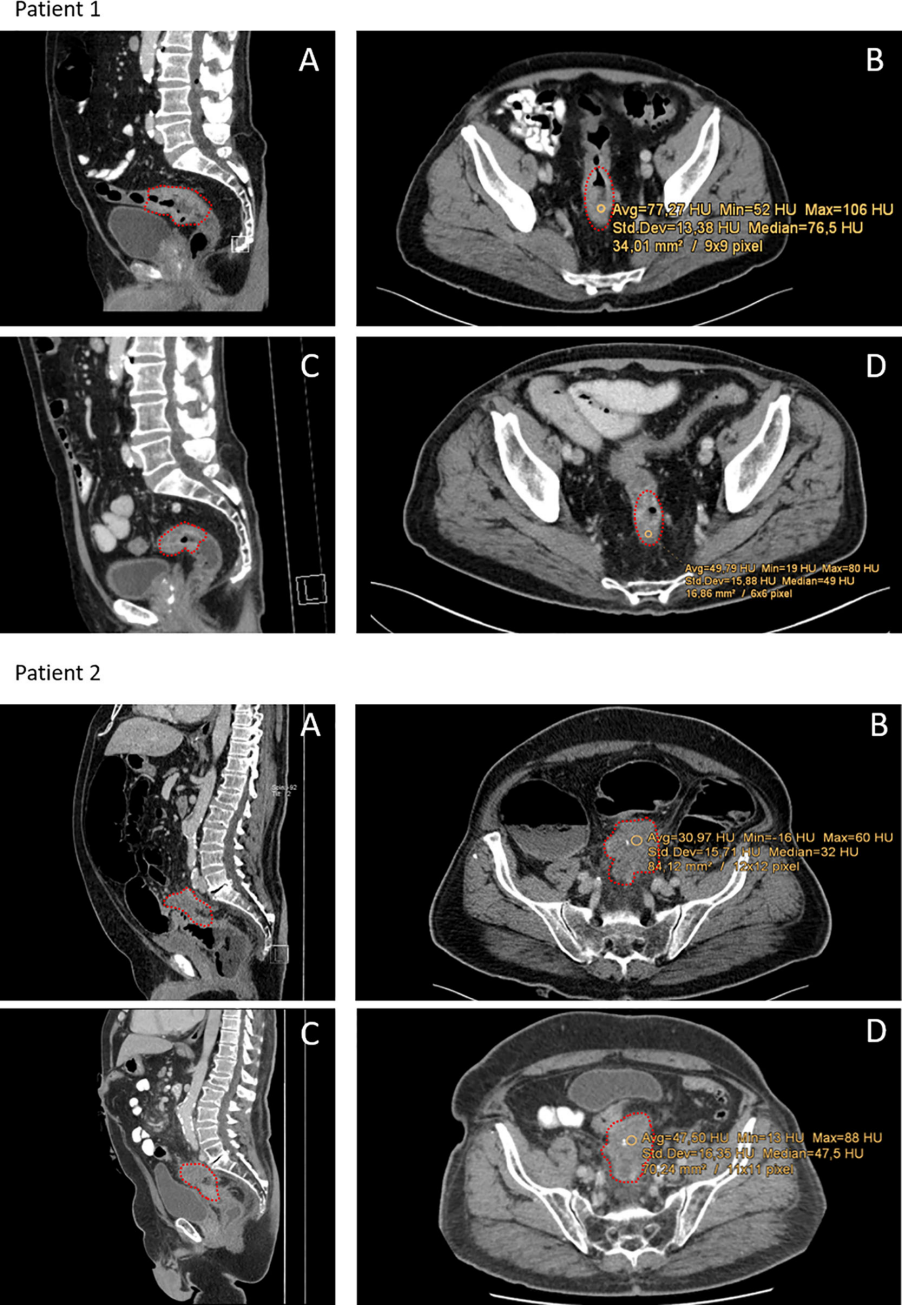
Representative CT-scans of patients with different responses towards neoCRT. Red dots indicate the tumor region. Patient 1: Patient with complete pathological response (regression grade 4 according to Dworak). (A, B) Show pre-neoCRT CT-scans in sagittal and axial profile. (C, D) Show CT-scans post-neoCRT. Median HU of the tumor was 76.5 HU pre- and 49 HU post-neoCRT. Patient 2: Patient with progressive disease and regression grade 1 according to Dworak and 80% viable tumor cells. (A, B) Show pre-neoCRT scans, (C, D) post-neoCRT CT-scans. Median HU increase from 31 to 48.
